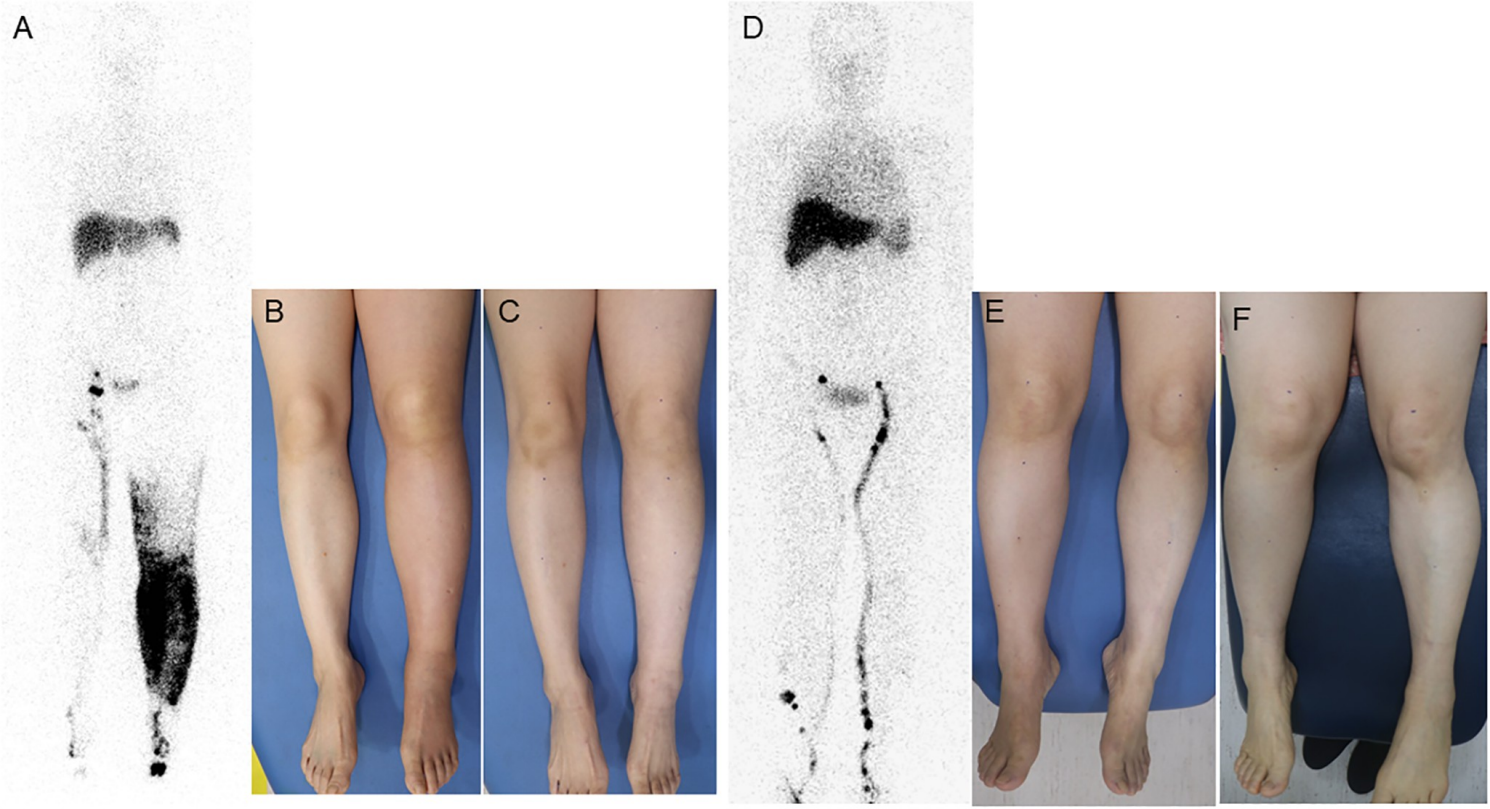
Representative case of LEL patients who underwent LVA based on lymphoscintigraphic findings. A 54-year-old woman with a history of endometrial cancer underwent radical abdominal hysterectomy (RAH) and bilateral pelvic lymph node dissection (BPLND) She had lymphedema in her left leg for the 9 months and had no history of cellulitis (A-C). (A) Lymphoscintigraphy revealed large extent of dermal backflow (DBF) on her left leg without visualization of regional lymph nodes. The main lymphatic vessels exhibited a lower-leg restricted pattern. (B) Preoperative photograph of lower extremities. The left leg presented with Stage II lymphedema with redness and pitting edema. The preoperative LELi was 446.8. (C) Postoperative photograph at 6 months. Following LVA on the left leg with three anastomoses at the saphenous, medial calf, and lateral calf, improvement in LELi was observed with enhanced redness and reduced pitting edema. After LVA, she had significant changes in LELi, with a LC rate of -13.48%. A 54-year-old woman with a history of endometrial cancer also underwent RAH and BPLND. She had lymphedema in her right leg for the 3 months and had no history of cellulitis (D-F). (D) Lymphoscintigraphy revealed no DBF with a trunk pattern of lymphatic vessels and visualized regional lymph nodes in her right leg. (E) Preoperative photograph of lower extremities. The right leg exhibited Stage II lymphedema with mild redness and pitting edema. The preoperative LELi was 440.5. (F) Postoperative photograph at 5.5 months. Four LVA anastomoses were performed at the mid lower leg, proximal lower leg, and mid-thigh. While the redness improved, pitting edema persisted. The LELi did not show improvement, with a total LC rate of 7.11%.
